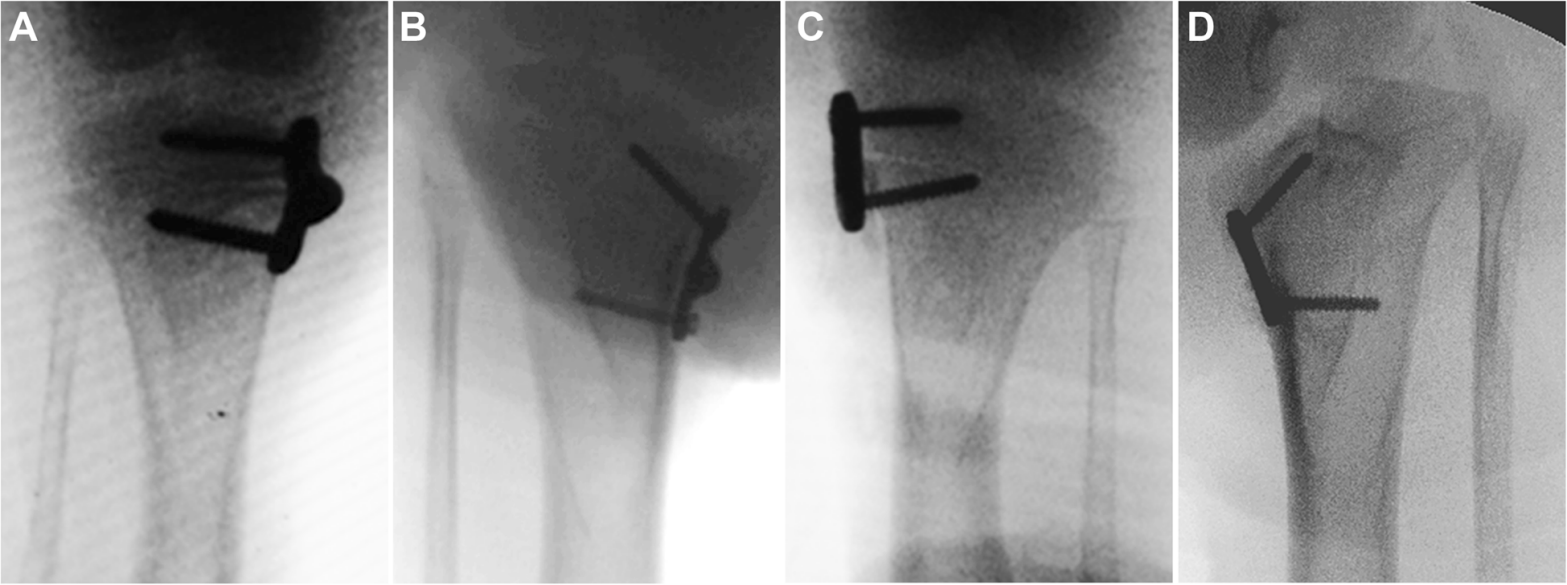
Standard anterioposterior radiographs of the tibia. (A) Immediately after correction with a hinged plate and screw system. (B) Eighteen weeks after operation. (C) Immediately after correction with a conventional plate and screw system. (D) Eighteen weeks after operation.
